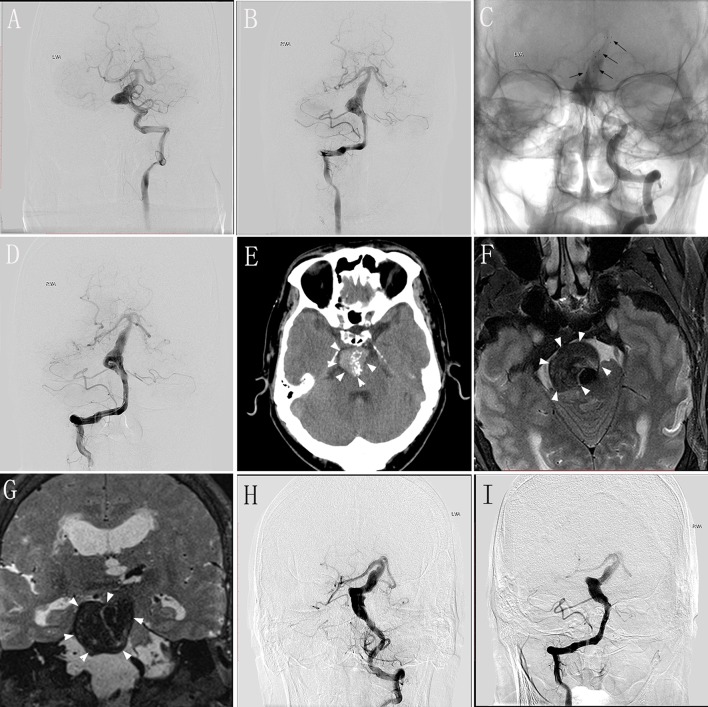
Images from a 48–50-year-old man with vertebrobasilar dolichoectasia (VBD) involving the basilar artery (BA) (case 5). (A,B) Preoperative digital subtraction angiography (DSA) of the left vertebral artery (VA) (A) and right VA (B) showing the involvement of the BA. (C) Unsubtracted view of the left VA immediately post-treatment showing the location of the stents (black arrows). (D) Immediately-post-treatment angiography of the right VA showing no change in the diameter of the diseased artery and obvious stasis of the contrast agent. (E) Follow-up computed tomography 5 months post-treatment showing a mass effect with compression of the brainstem (white arrowheads). (F,G) Transverse (F) and coronal (G) magnetic resonance images 24 months post-treatment showing severe brainstem compression. (H,I) Follow-up DSA of the left VA (H) and right VA (I) at 24 months showing the deterioration in the length and diameter of the diseased artery.
